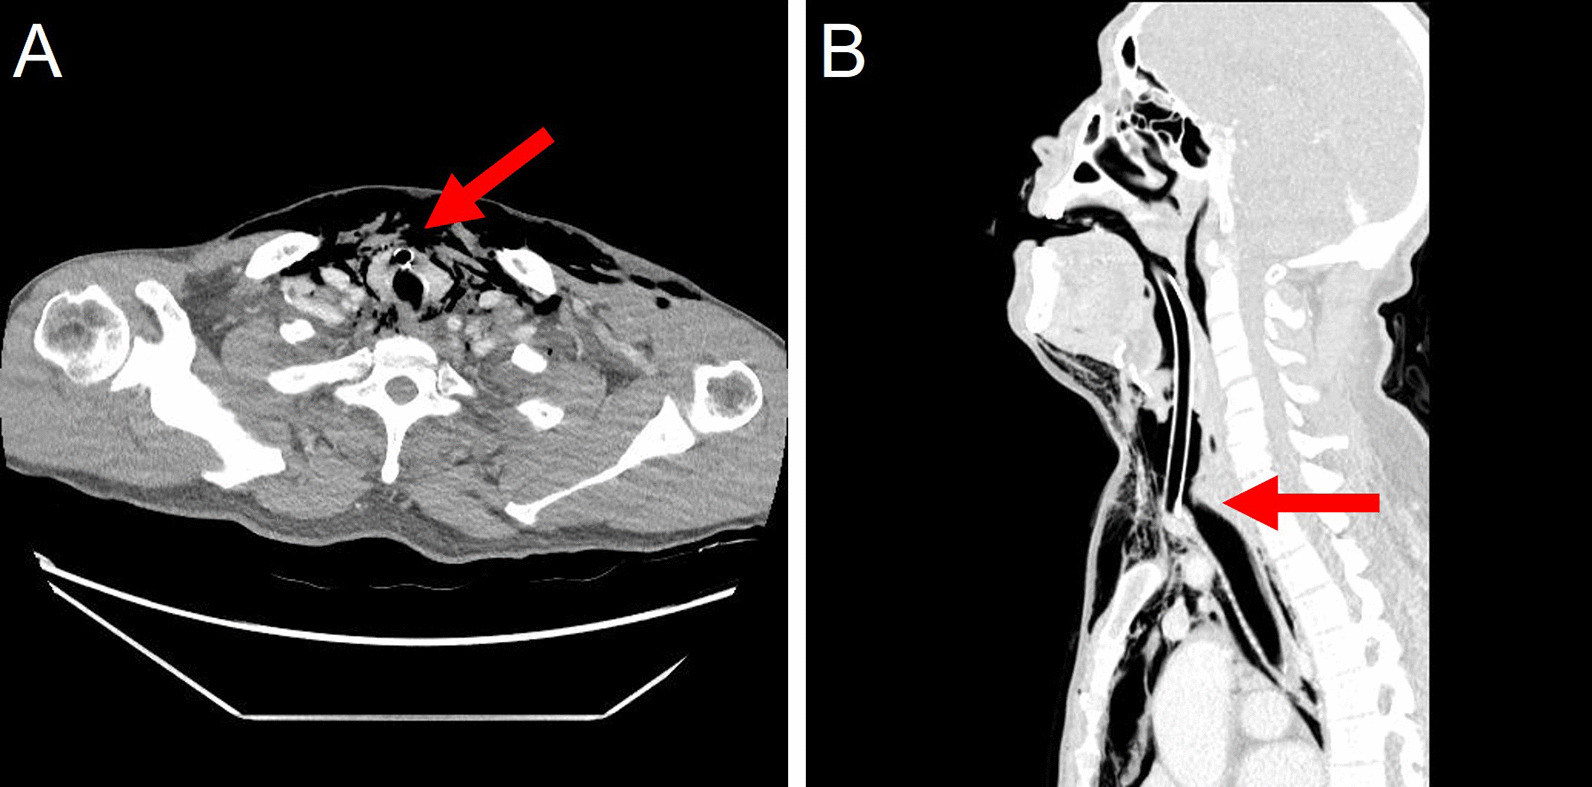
Computed tomography finding during the initial diagnostic period. a Intubation tube protruding toward the anterior side of the fractured trachea (red arrow); transverse view. b Intubation tube protruding toward the anterior side of the fractured trachea (red arrow); sagittal view
